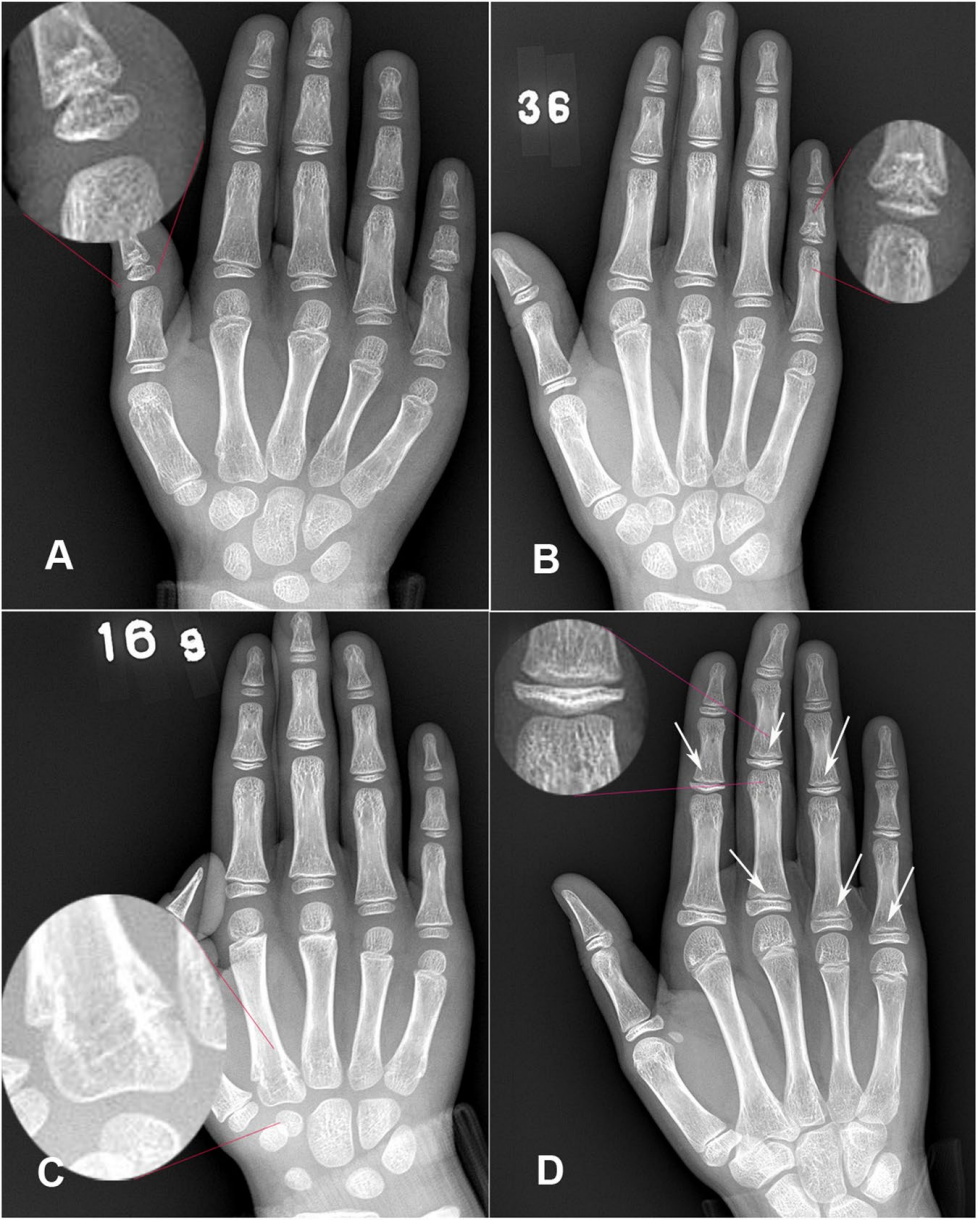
(A) Is an X-ray image of the right hand of a 7-year-old girl with changes of the thumb: cone-shaped epiphysis is embedding the metaphysis of the thumb singly. (B) Is a radiological image of the right hand of an 8-year-old girl with variation in the little finger: cone-shaped epiphysis was embedding metaphysis badly in middle phalanx of little finger. All the other parts were normal. (C) Is a radiological image of the right hand of an 8-year-old boy with the second metacarpal-phalangeal variation: Two epiphyses are connected to the second metacarpal. All the other parts are normal. (D) Is a radiological image of the right hand of a 10-year-old girl with cystic changes: cystic changes in index finger, middle finger, ring finger and little finger (yellow arrows).
